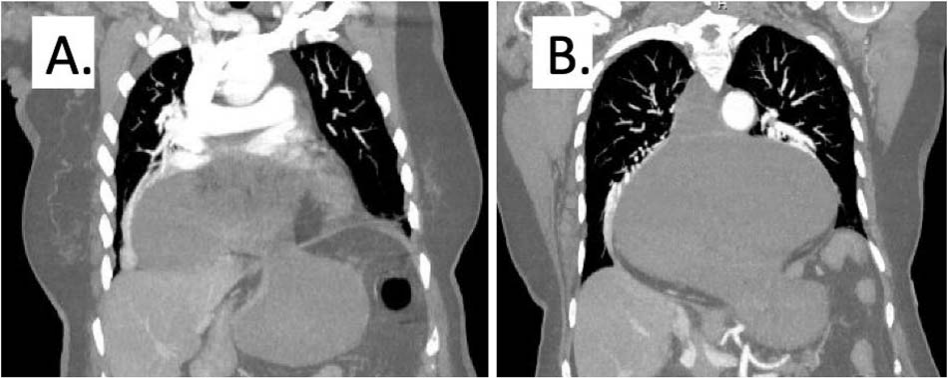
Representative computed tomography image demonstrating gastric distention and area of volvulus (A) and extent of hiatal hernia (B).
